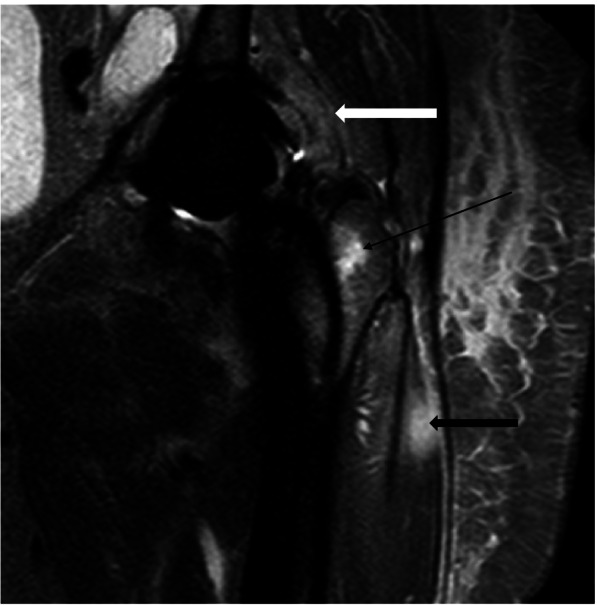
Coronal MARS-STIR-MRI shows layering (white arrow) and hyperintensity of the synovium which is highly suggestive of an infectious process. Femoral bone marrow (black thin arrow) and muscle edema (black thick arrow) indicates periprosthetic stress reaction
